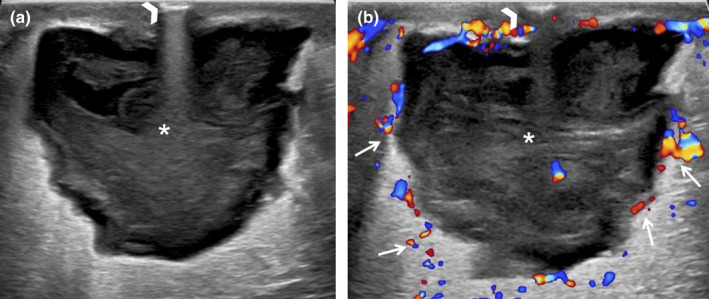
25‐year‐old woman with a right nipple piercing presented with periareolar right breast pain and a lump. Ultrasound evaluation was performed with a L4‐18 MHz transducer with harmonics and the colour gain set to 2.5 cm/s. Greyscale image showed a large complex collection (asterisk) with posterior acoustic through transmission (a). Colour image showed marker peripheral hyperaemia and vascularity (arrows) (b). Both images included linear echogenicity with reverberation artefact from the nipple piercing (chevron).
